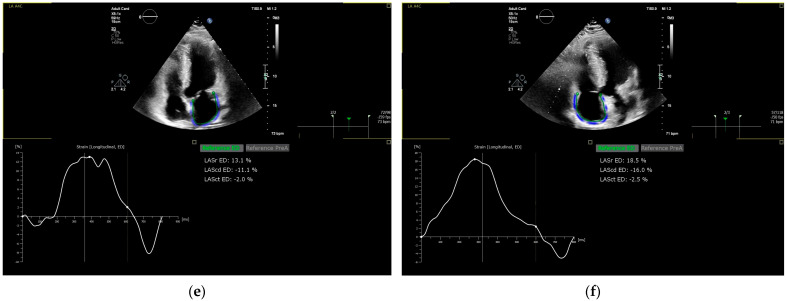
Clinical case of a patient diagnosed with HOCM, who experienced paroxysmal AF episodes, showing left and right atrial remodeling and elevated filling pressures, both predisposing factors to AF development. The images present echocardiographic measurements associated with AF onset in patients with HOCM. Images (a–f) were obtained from the institutional database and were anonymized. (a) Increased LA antero-posterior diameter (≥45 mm). (b). Marked dilation of the LA area (≥28 cm^2^) and volume. (c,d). Elevated left ventricular filling pressures (E/e′ ratio = 17.7). (e,f). Alterations of both left and right atrial strain.
